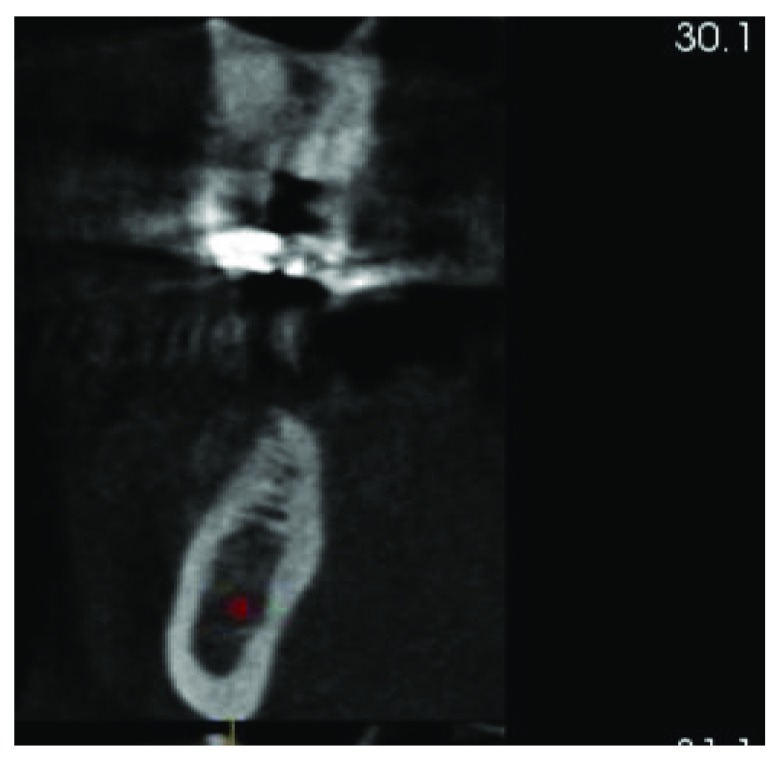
CBCT showing insufficient bone width #46.
